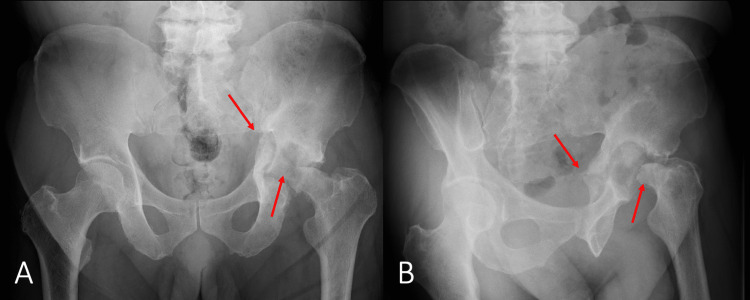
Plain radiographs of the pelvis at our hospital A) Frontal radiograph; B) Left oblique radiograph Pseudarthrosis of the transverse-posterior wall acetabular fracture and destruction of the femoral head have been demonstrated (red arrows)
